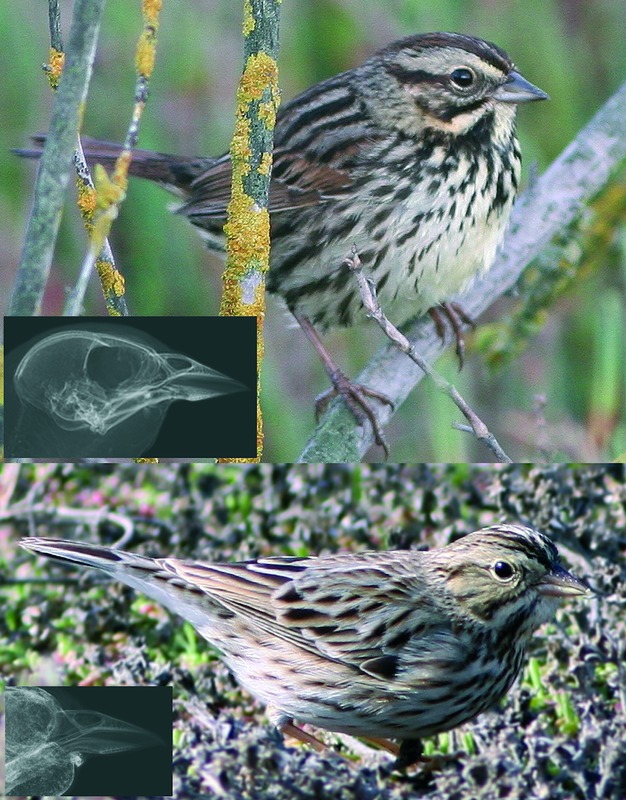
Photographs and radiographs of Alameda song sparrow (top) and Belding's savannah sparrow (bottom). Photographs courtesy of John Sterling.
